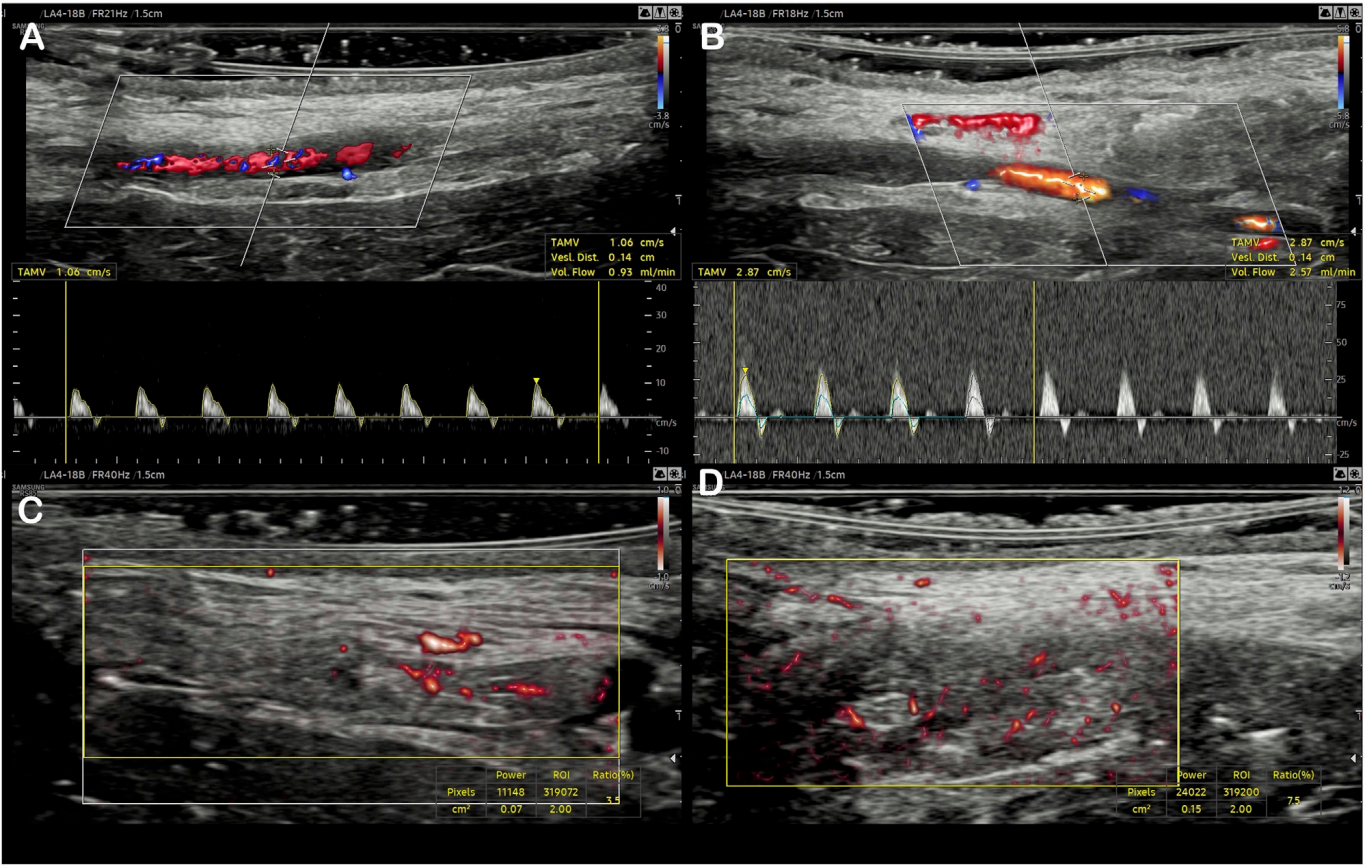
Evaluation of the initial arterial flow using color Doppler and pulsed Doppler (A), then after femoral artery clamping (C). A similar presentation of the Vascularity Index for the same flap using MV-Flow, at baseline (B), and after flow increase (D).
